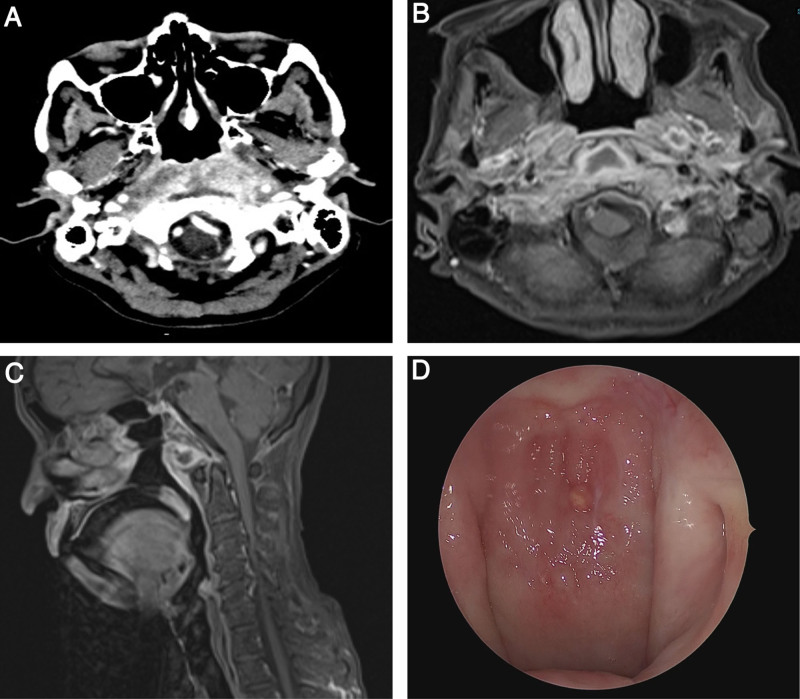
(A) Axial CT scan showing heterogeneous retropharyngeal soft tissue enhancement at the level of the skull base and nasopharynx and a small abscess (0.7 × 2.3 cm) anterior to the clivus with bony erosion. (B) Post-contrast T2-weighted axial MRI scan showing multiloculated collections in the retropharyngeal (1.2 × 1.6 × 1.4 cm) and left parapharyngeal (0.8 × 1.0 × 0.9 cm) regions with peripheral enhancement, suggestive of abscesses. (C) Post-contrast T2-weighted sagittal MRI scan showing the retropharyngeal abscess and clival osteomyelitis. (D) Endoscopic view of the midline nasopharyngeal mass. CT = computed tomography.
